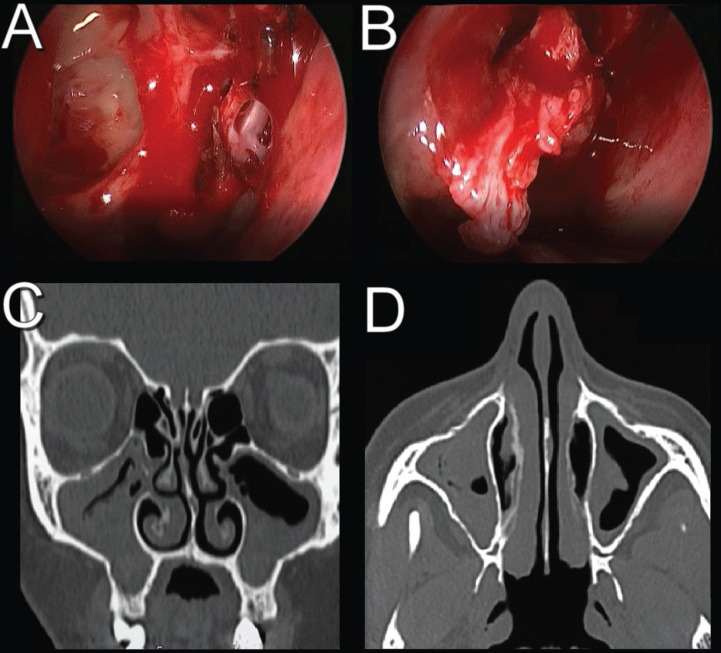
Initial complementary exams. A and B) Sequence of trans-surgical images of transnasal endoscopy; C) Coronal section of computed tomography; D) Axial section of computed tomography.
